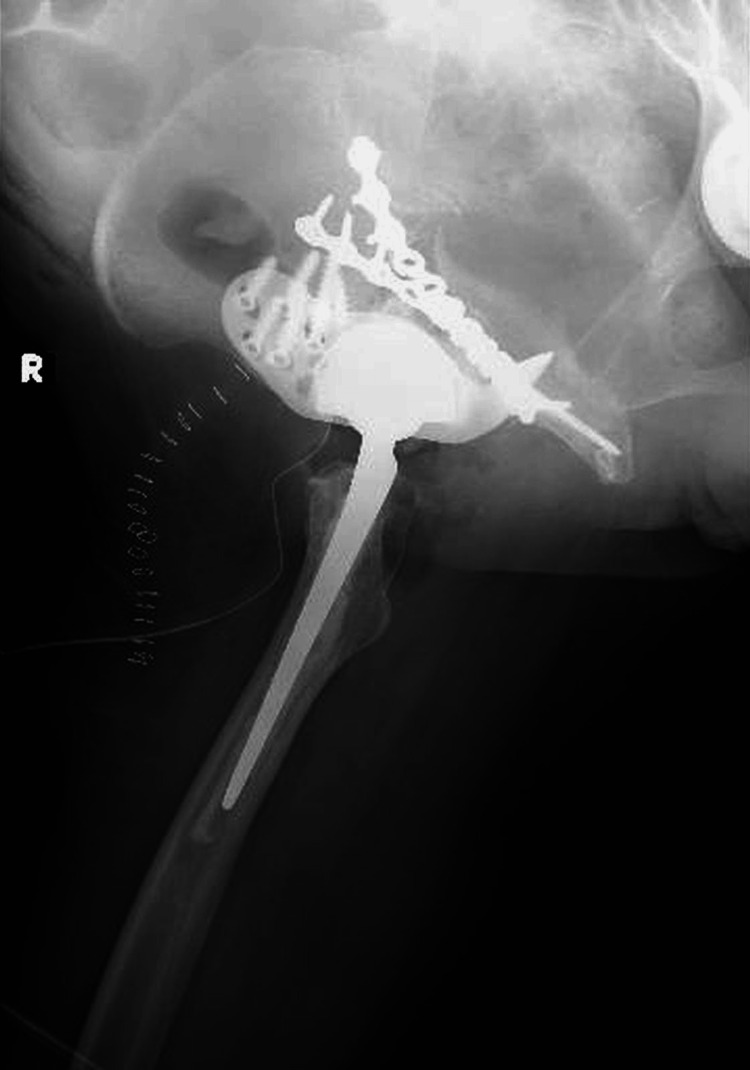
Postoperative plain radiograph of the pelvis (lateral view). Plate fixation was performed from both the anterior and posterior aspects. The acetabulum was reconstructed using a cage, and the femoral stem was revised with a cemented stem.
